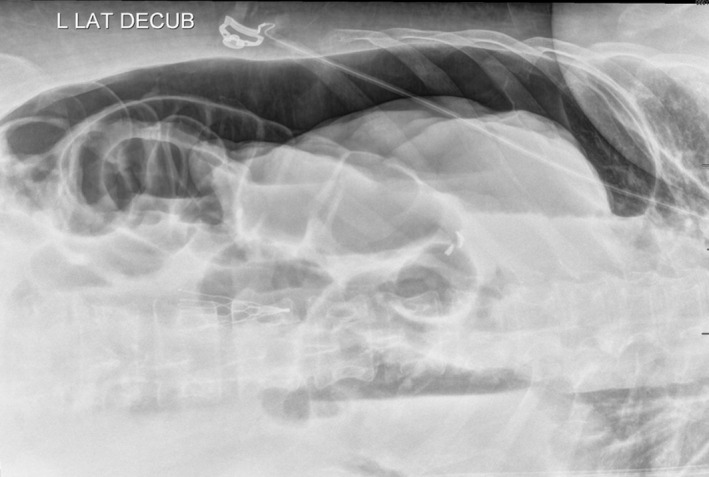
Abdominal radiograph in left lateral decubitus position
